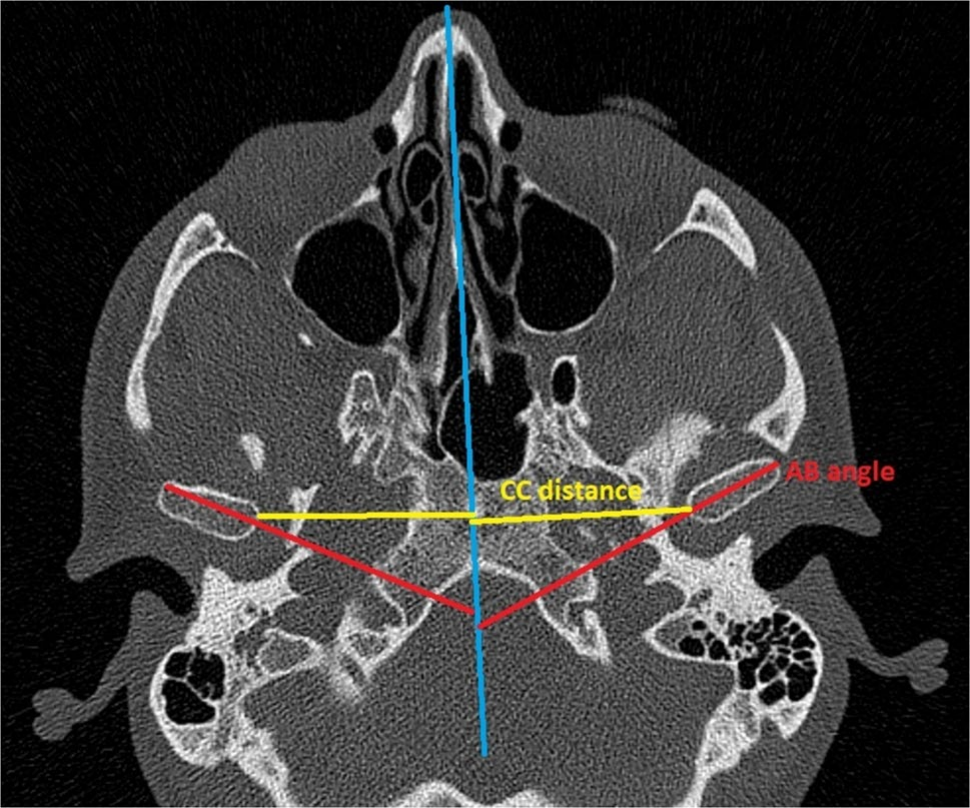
Axial CT condylar position parameters
